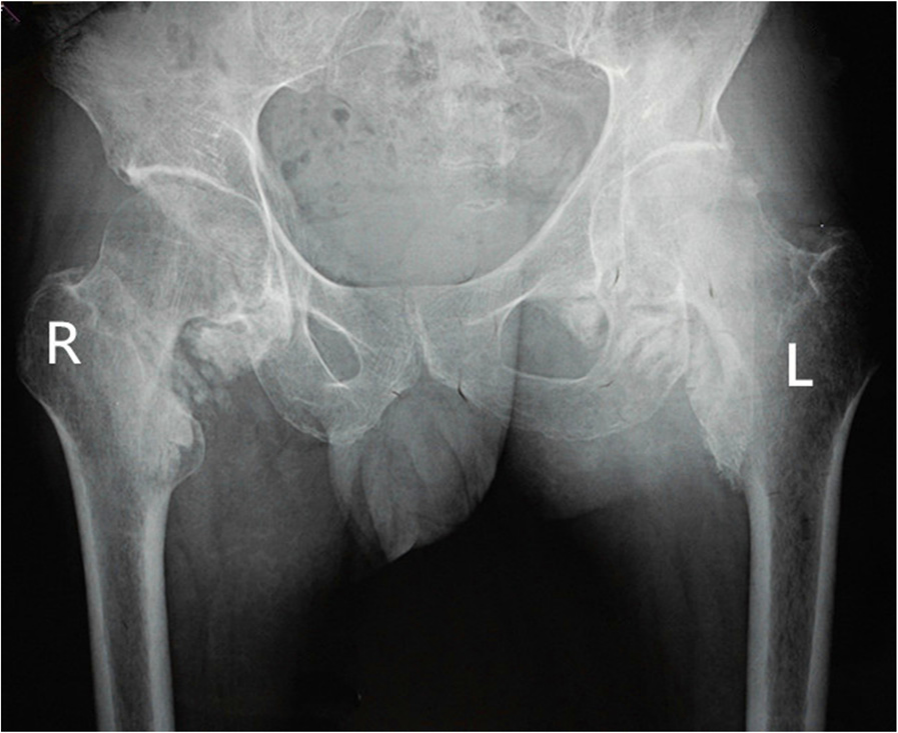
Preoperative radiographs of the pelvis shows para-articular HO on the interior aspect of both the femoral necks.
